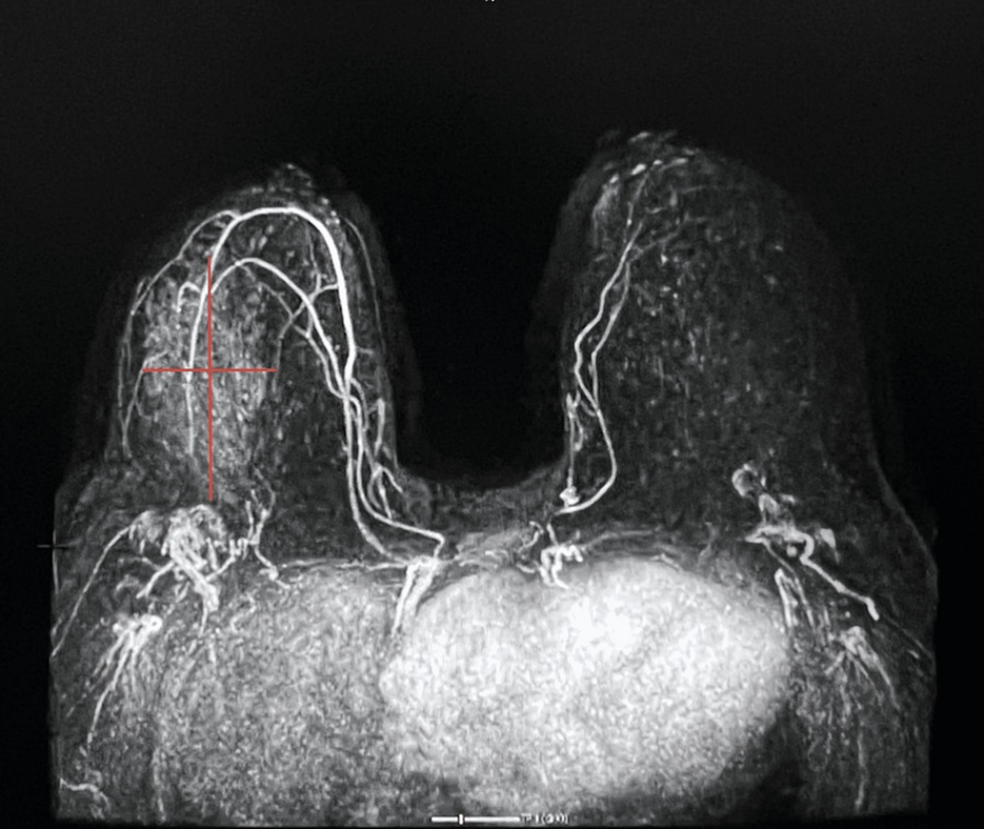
Axial MRI of the breast showing a 9x10.5x4.6 cm non-mass-like enhancement in the right breast
